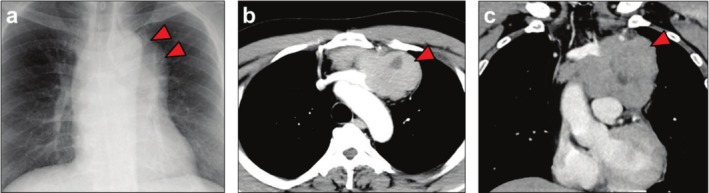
Imaging findings at diagnosis. (a) Chest radiograph. (b, c) Contrast‐enhanced chest computed tomography. (b) Axial images and (c) coronal images. Arrowheads indicate the tumor.
